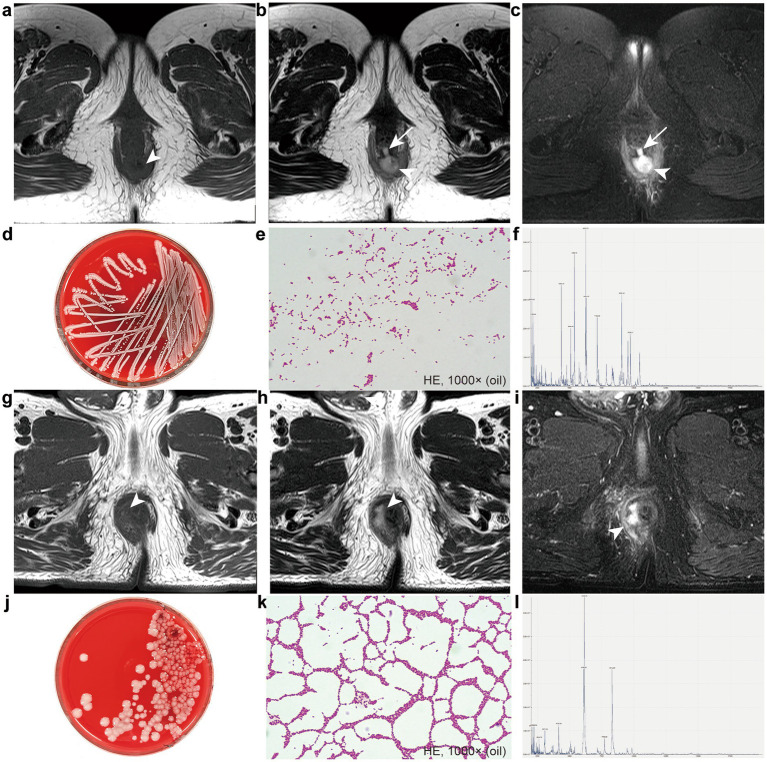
MRI findings and pathogen distribution in patients with perianal abscesses. (a–f)
